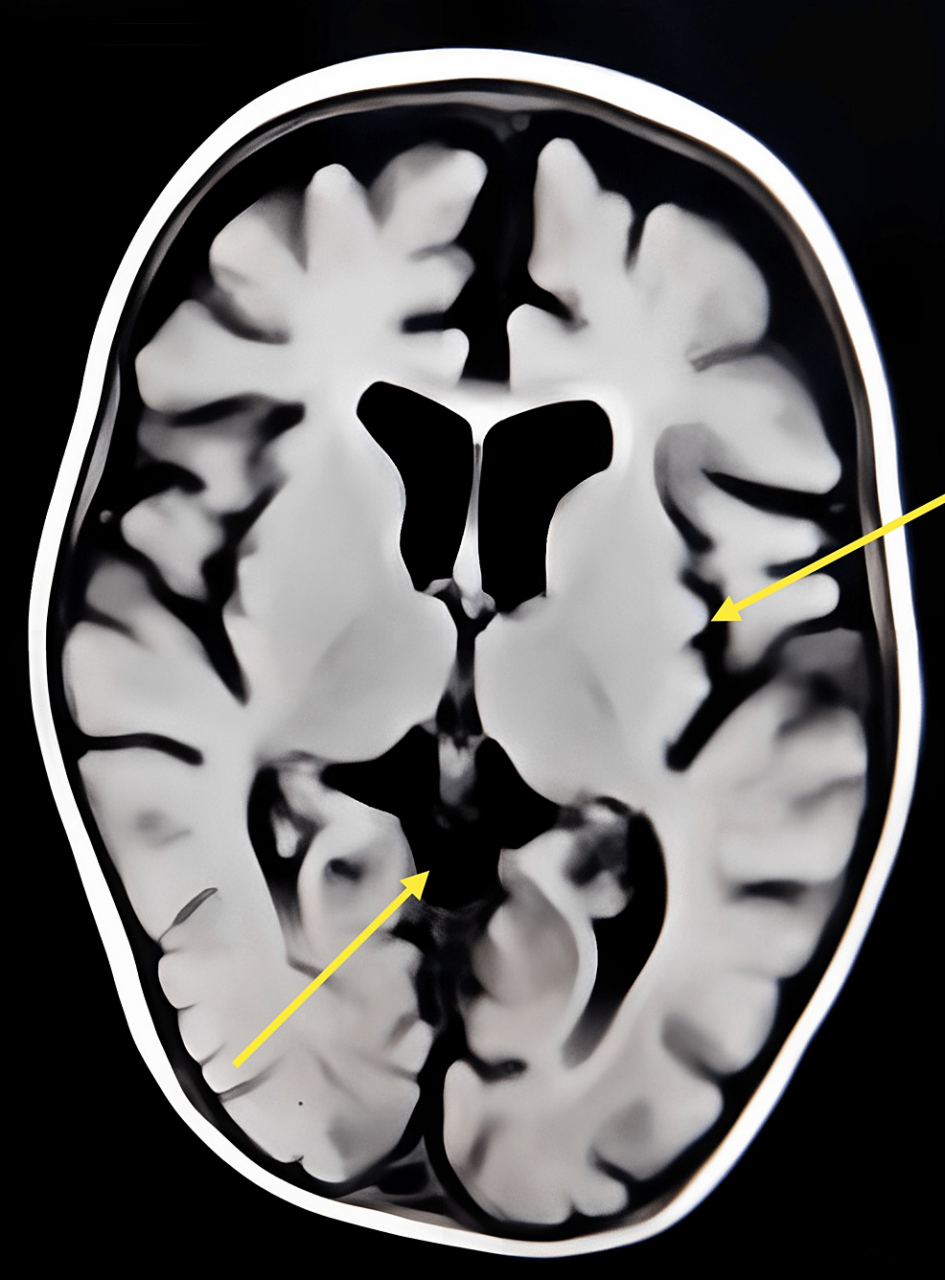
MRI showing cerebral atrophy and ventricular prominence.
